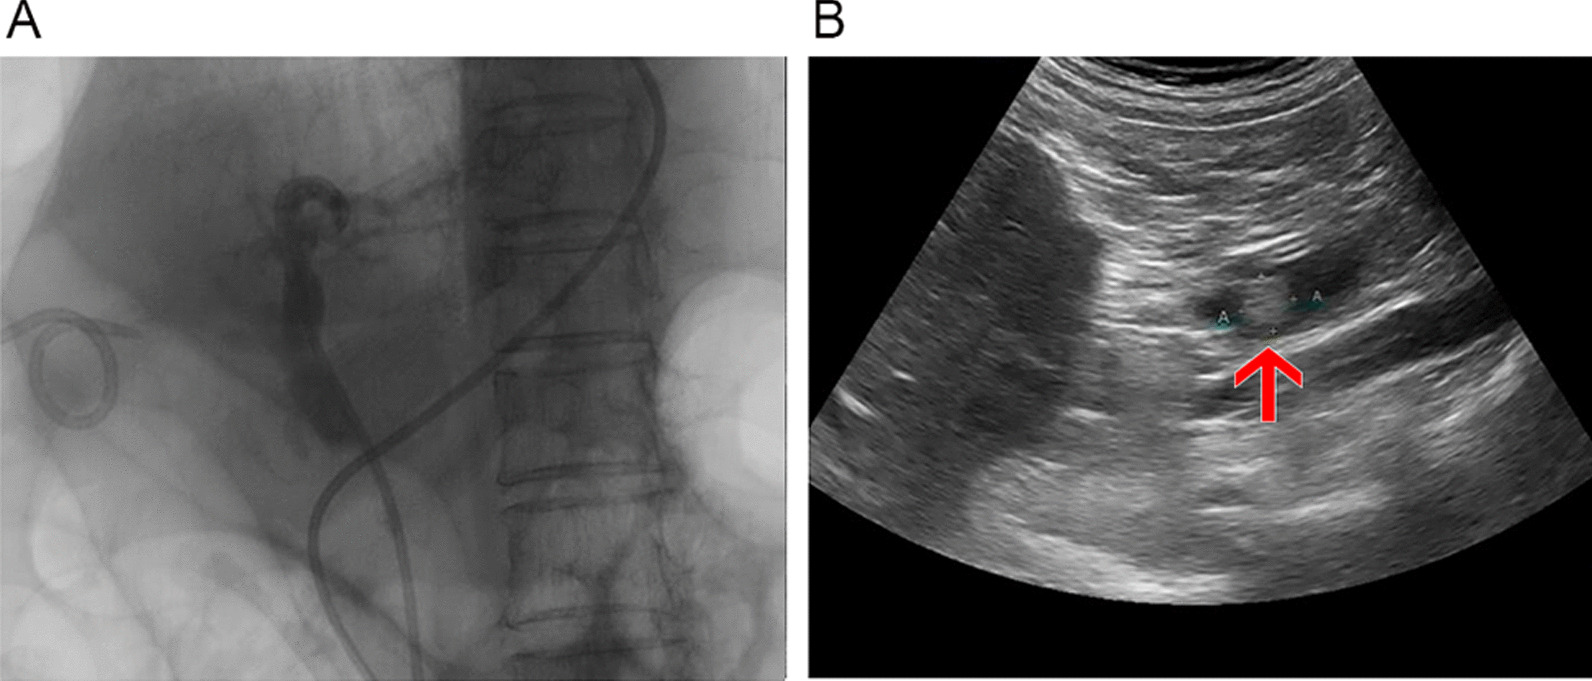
Comparison between occlusion cholangiogram and ENBD saline injection in ultrasounds in a 78-year-old women. A. There are no significant stones found in occlusion cholangiogram. B. Remnant stone is found by ENBD saline injection ultrasound
